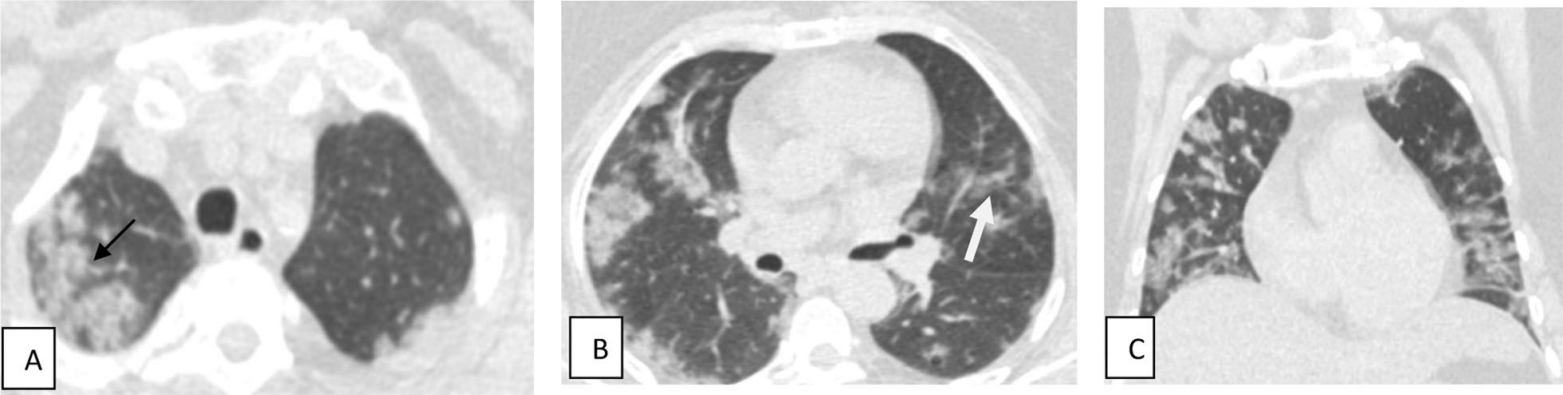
Female patient 46 years old presented by cough, dyspnea and fever. Axial (A, B) and coronal (C) CT images show bilateral multifocal ground-glass opacities (white arrow); areas of consolidation and areas of interstitial thickening giving crazy-paving appearance (black arrow) are seen involving both lung fields with peripheral/subpleural predominance (moderate to severe severity)
